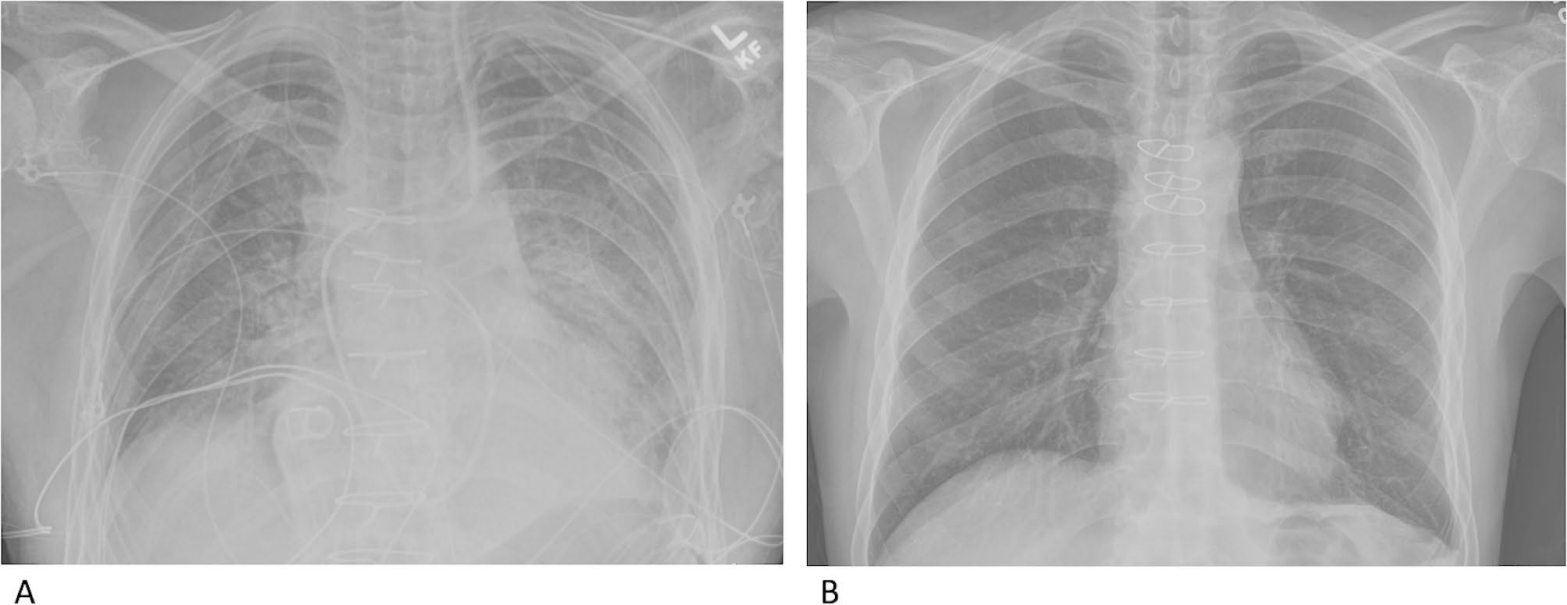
Postoperative chest xray at postoperative day two (A) and three months following heart-lung transplant (B)
